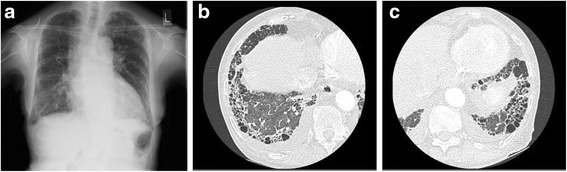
a Chest radiography 1 month before AVR. Chest radiography showed a cardiothoracic ratio of 63 % and bilateral infiltration shadow. b CT findings of the right lower fields of the lung. c CT findings of the left lower fields of the lung. CT indicated usual interstitial pneumonia pattern. *AVR* aortic valve replacement, *CT* computed tomography
